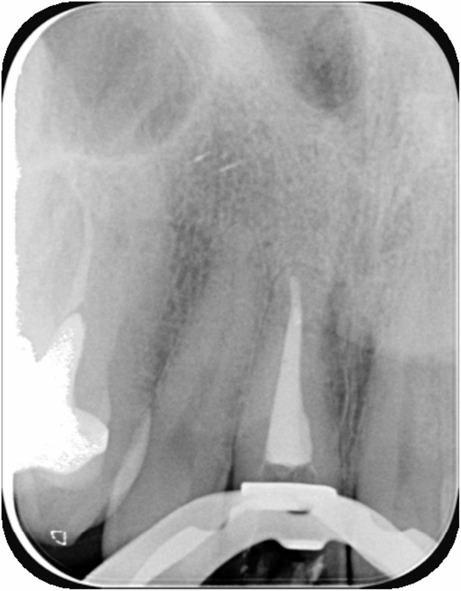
Post operative periapical X-Ray showing the root canal obturation
